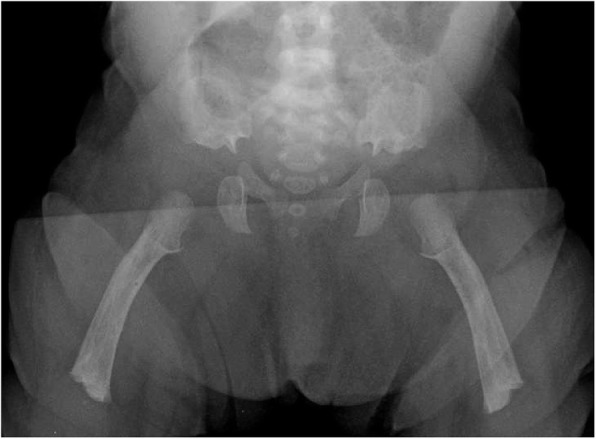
Anteroposterior radiograph of the pelvis and femora in an infant with achondroplasia. Characteristic findings include a squared-off pelvis, horizontal acetabula, very narrow sacrosciatic notch, characteristic proximal femoral radiolucency, and short and robust femora
